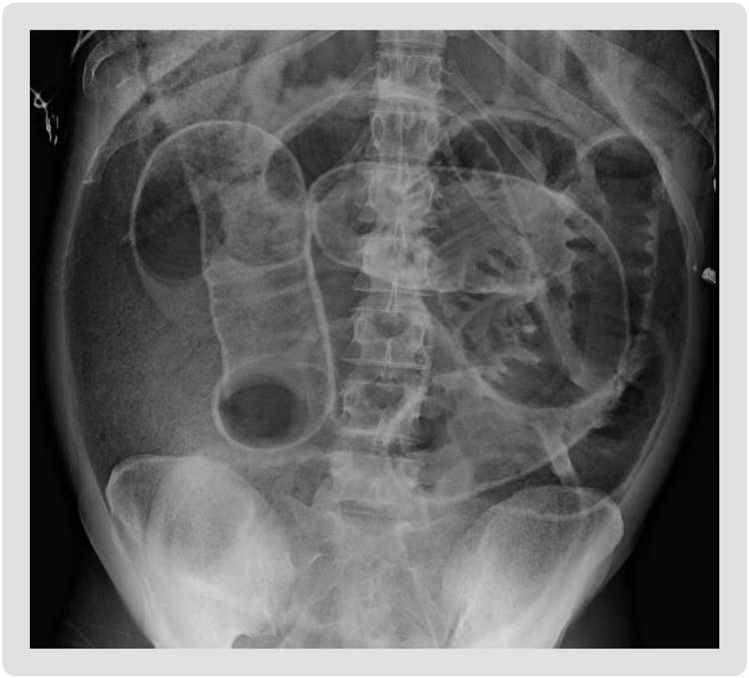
Supine abdominal plain film on admission demonstrating Rigler’s sign, bowel loops outlined by air, increasing suspicion for large volume pneumoperitoneum.
